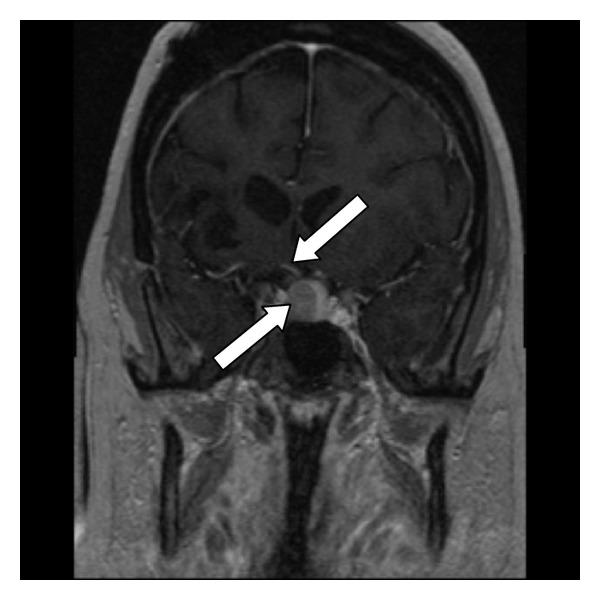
MRI of pituitary (coronal view) (arrows showing the macroadenoma and stalk deviation).
